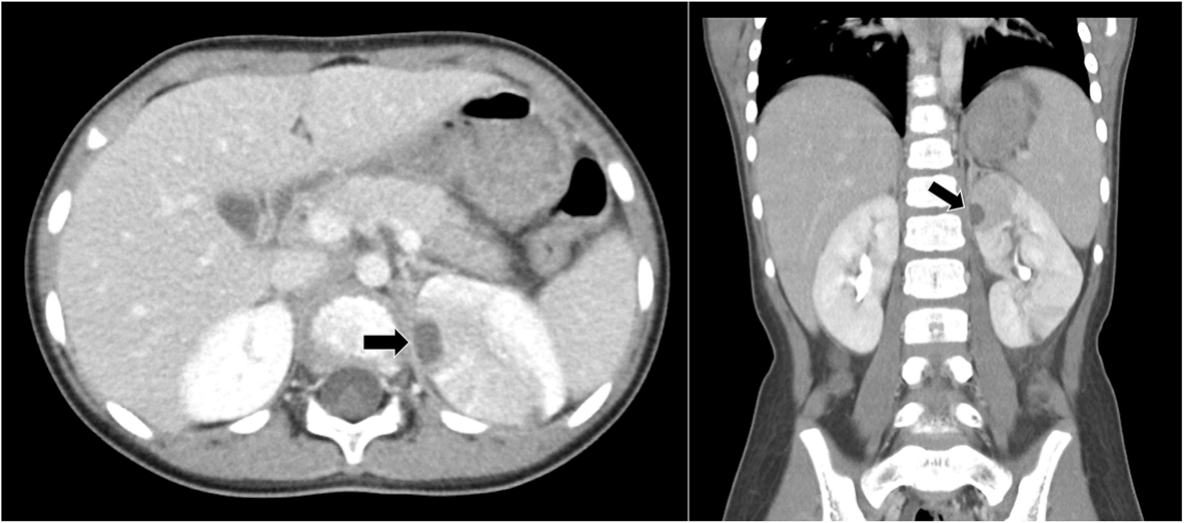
Computed tomography (CT) scan performed on day 3 of admission shows a left superior renal abscess (13 × 9 × 10 mm, arrow). Low-density areas in the superior and anterior poles of the left kidney suggesting acute focal bacterial nephritis are seen
